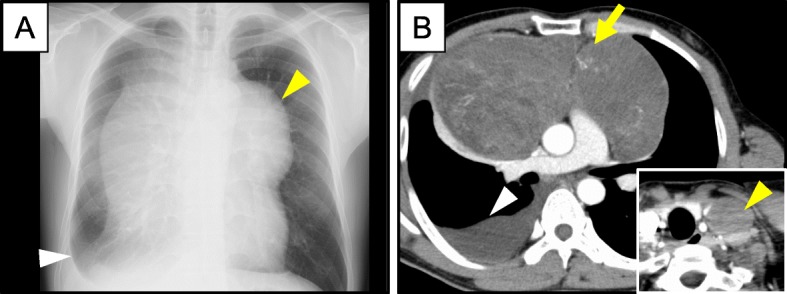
Chest X-ray and CT scan. a Chest X-ray reveals a well-circumscribed bilateral hilar mass approximately 20.5 cm in maximum dimension (yellow arrowhead), and dullness of the right costal pleural angle (white arrowhead). b CT scan reveals a 19.5 cm × 10.8 cm heterogeneously enhancing anterior mediastinal mass (yellow arrow) and a 4.3 cm left cervical mass (yellow arrowhead), and right pleural effusion (white arrowhead)
